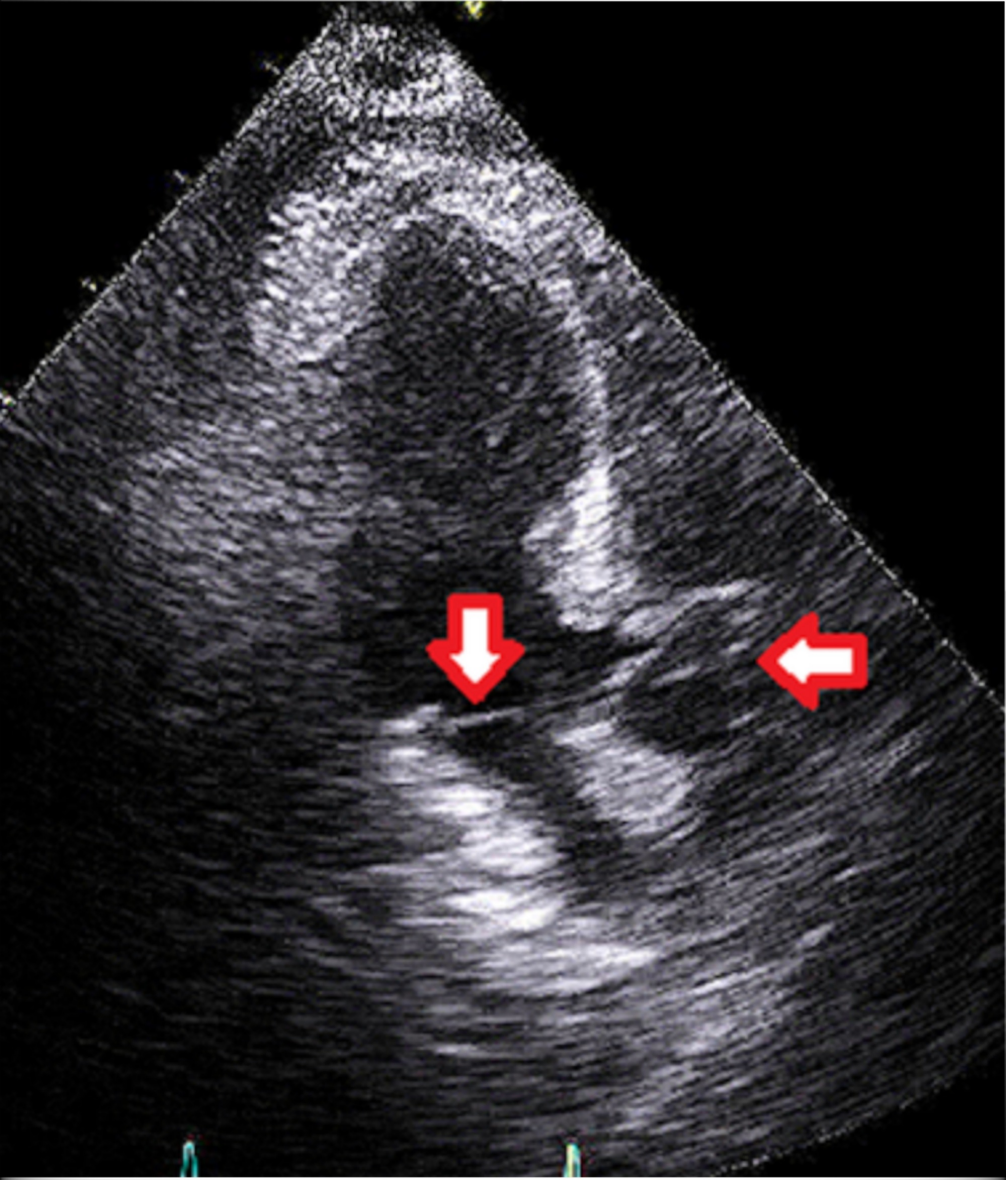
Transthoracic Echocardiogram (apical three-chamber view) Downwards facing red arrow shows the mitral valve, without any vegetations. Leftwards facing red arrow shows the aortic valve, also without vegetations.
